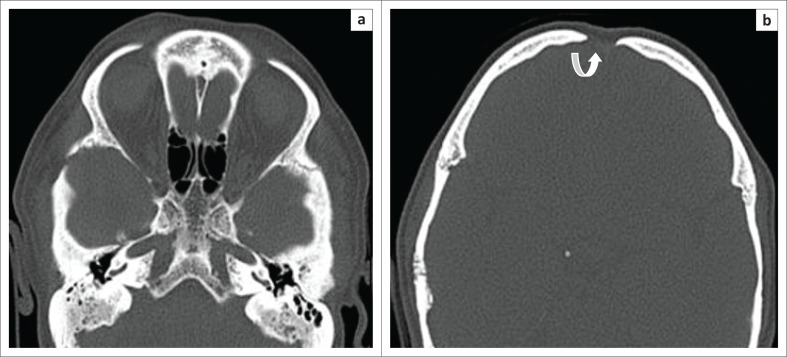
Axial-computed tomography section at the level of skull base (a) shows under-pneumatised mastoids, non-pneumatised sphenoid sinuses and superiorly (b) shows an open metopic suture (curved arrow).
